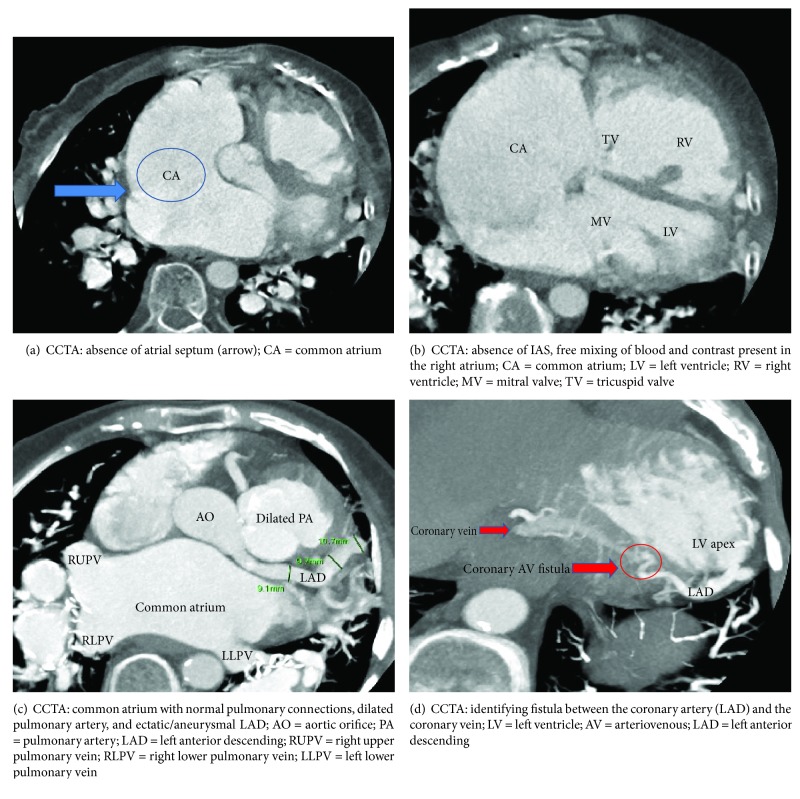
Cardiac computed tomography angiography images. (a) Absence of the atrial septum (arrow). (b) Absence of interatrial septum showing free mixing of blood and contrast present in the atrium. (c) Common atrium with normal pulmonary connections, dilated pulmonary artery, and ectatic/aneurysmal left anterior descending artery. (d) Identifying fistula between the coronary artery and the coronary vein.
